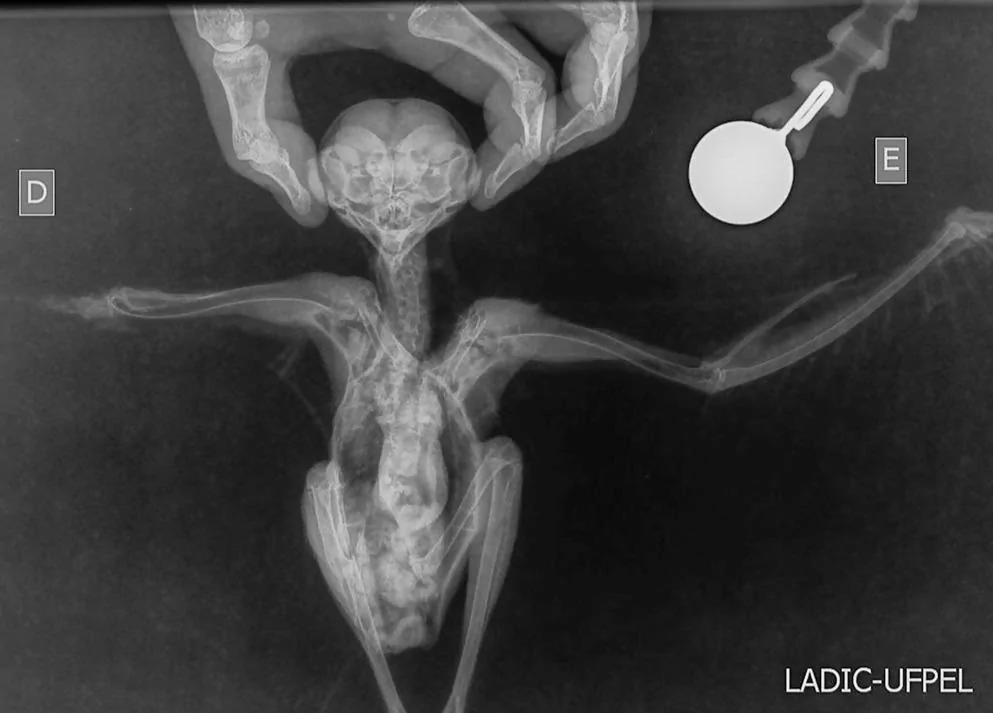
Ventrodorsal whole-body radiographic projection of *Athene cunicularia*. Image obtained at the Diagnostic Imaging and Cardiology Laboratory (LADIC/UFPel). In the right thoracic limb (R), complete agenesis of the radius, ulna, and distal structures is observed. In the left thoracic limb (L), partial absence and thinning of the distal radius are evident. Bilateral absence of all skeletal structures distal to the forearm (carpus, metacarpus, and phalanges) is noted. The absence of a bone callus supports the exclusion of a previous traumatic etiology
